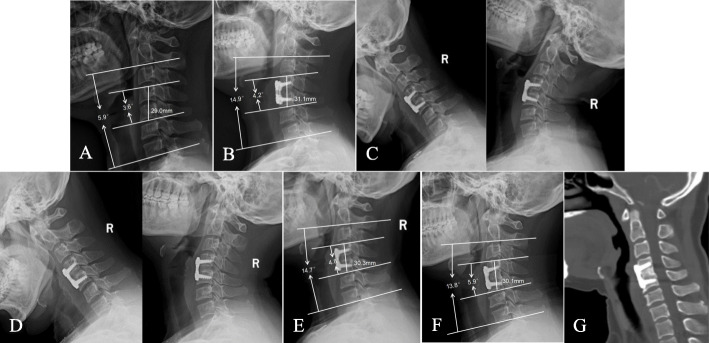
A 48-year-old female patient underwent single-level ACDF due to C4/5 disc herniation. A mixture of bone dust and morselized bone was used as an n-HA/PA66 cage-filling material during the operation. A Preoperative lateral radiograph of the cervical spine showing narrowing of the C4/5 disc space. B Postoperative lateral radiograph showing that the n-HA/PA66 cage increased the FSH, SSA, and C2–7 lordotic angle. C, D Flexion-extension lateral radiographs showing fusion at the 3-month and 6-month follow-ups. E, F Lateral radiographs showing mild cage subsidence at the 1-year and final follow-ups; the FSH, SSA, and C2–7 lordotic angle remained stable. G Three-dimensional CT at the 28-month follow-up time showing grade 5 fusion
